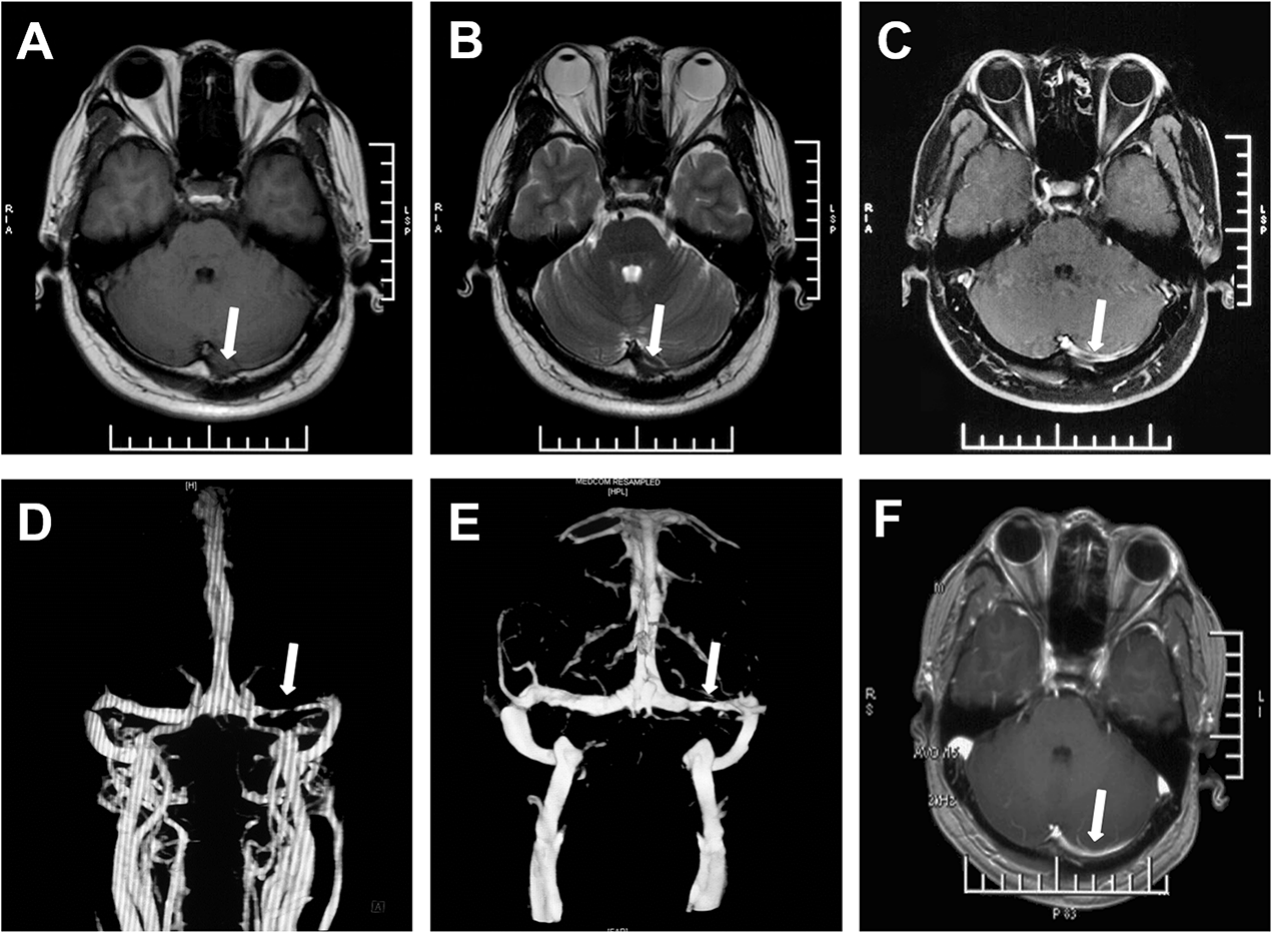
Double-track sign and sinus recanalization. A 42-year old male patient with left transverse sinus stenosis. Isointense on T1WI (A) and hypointense on T2WI (B) demonstrated an acute stage of thrombus. Double-track sign (arrow) was showed on axial Gd-enhanced T1WI (C). Transverse sinus stenosis was confirmed on MRV which showed a linear signal of blood flow in the left transverse sinus on MRV (D) (Time from MRI to MRV was 3 hours). Follow-up scan after 2 weeks of anticoagulation therapy showed that the stenosis was ameliorated than before on MRV (E), and the double-track sign disappeared on axial Gd-enhanced T1WI after the recanalization (F) (Time from MRI to MRV was 6 hours).
